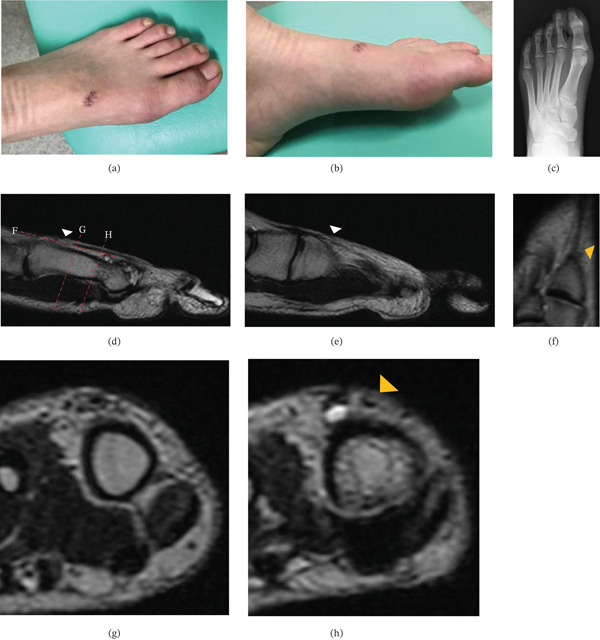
(a, b) Preoperative appearance showing a 1‐cm oblique laceration on the dorsomedial aspect of the midfoot. (c) Plain radiographs showing no osseous abnormalities. (d‐h) T2‐weighted magnetic resonance imaging demonstrating disruption of the extensor hallucis longus (EHL) tendon at the dorsal midfoot (d, e: sagittal; f: coronal; g, h: axial views). In the coronal and axial views, the extensor hallucis capsularis (EHC) is also identified as a thinner medial structure adjacent to the EHL (yellow arrowhead).
